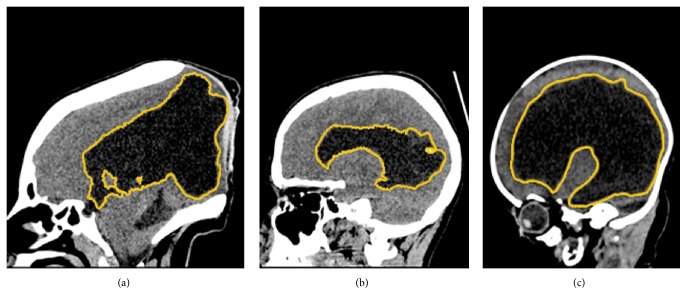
Variability in the size, shape, and distribution of CSF. Sagittal reconstructions from images of three different patients from our dataset that were treated for hydrocephalus. Examples include (a) a patient with encephalocele (treated surgically before the CT scan) and ((b) and (c)) patients with prematurity-associated intraventricular haemorrhage grade IV with bleeding extending into the brain tissue around the ventricles. Patients were treated with a ventriculoperitoneal shunt. Ventricular system boundaries are marked with a yellow line.
